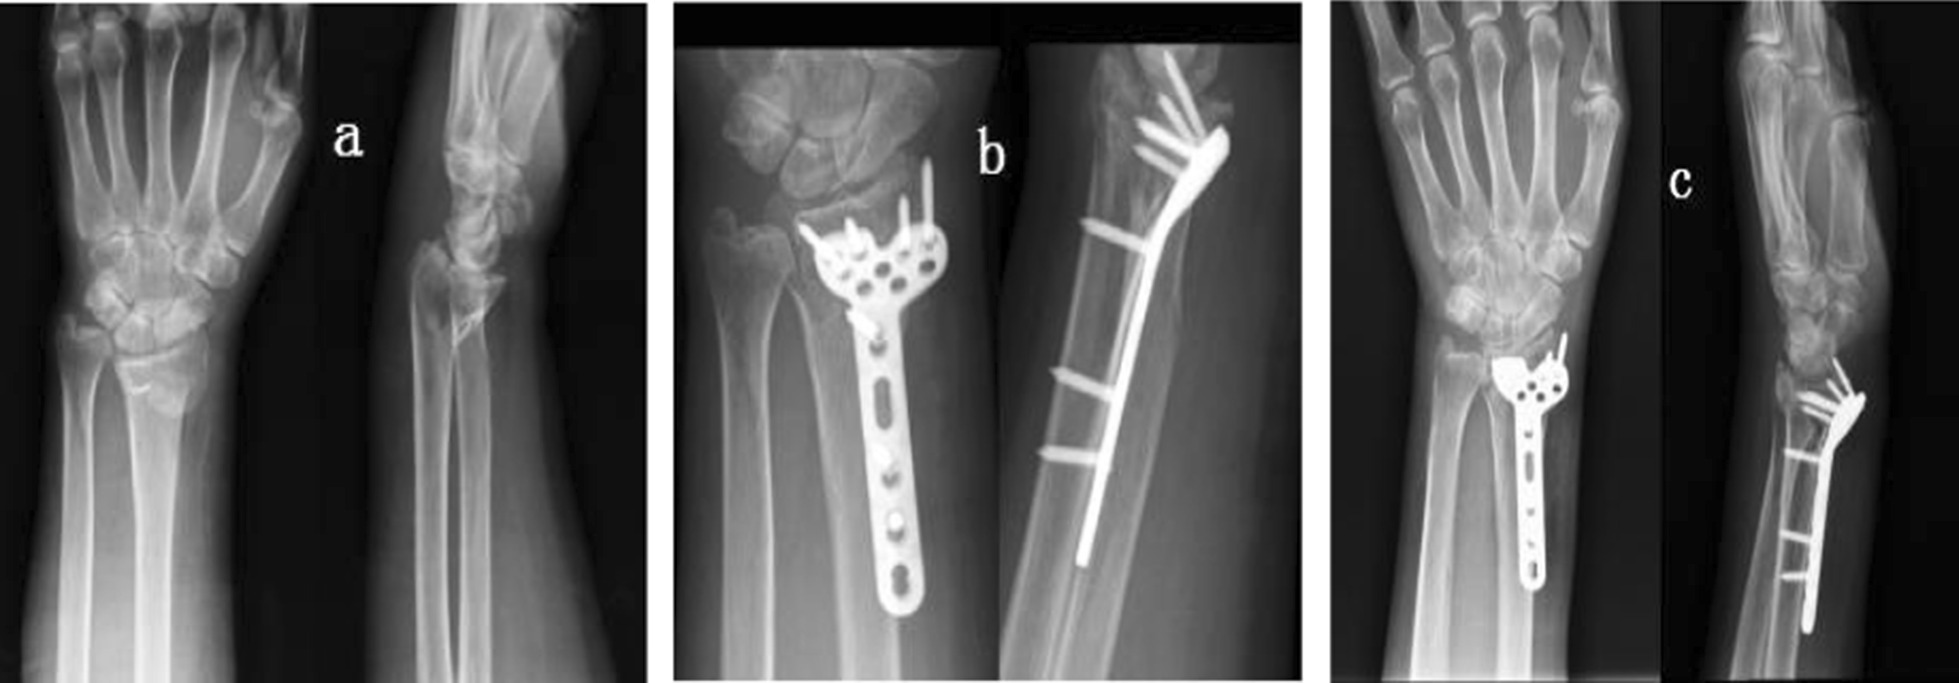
X-ray observations of Case 2. a Shows a preoperative X-ray showing a fracture of the right distal radius; b Shows the X-ray on the next day of surgery, showing the exact reduction and fixation of the fracture; c Shows the X-ray at 6 weeks after surgery, where the fracture line is still visible
